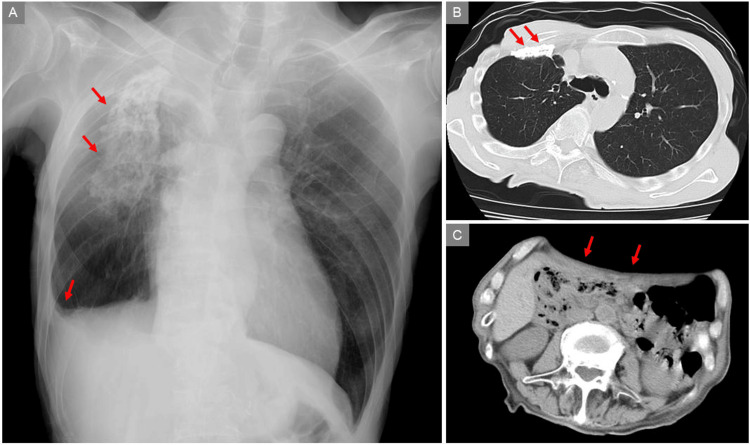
Chest radiography and computed tomography images Chest radiography showed calcifications in the right upper and middle lung fields and blunting of the right costophrenic angle (A, arrows). Computed tomography revealed calcifications along the anterior pleura without pleural effusion (B, arrows), and these findings were considered sequelae of prior pulmonary tuberculosis. A concave abdominal wall was also noted (C, arrows), suggesting malnutrition or loss of subcutaneous fat.
